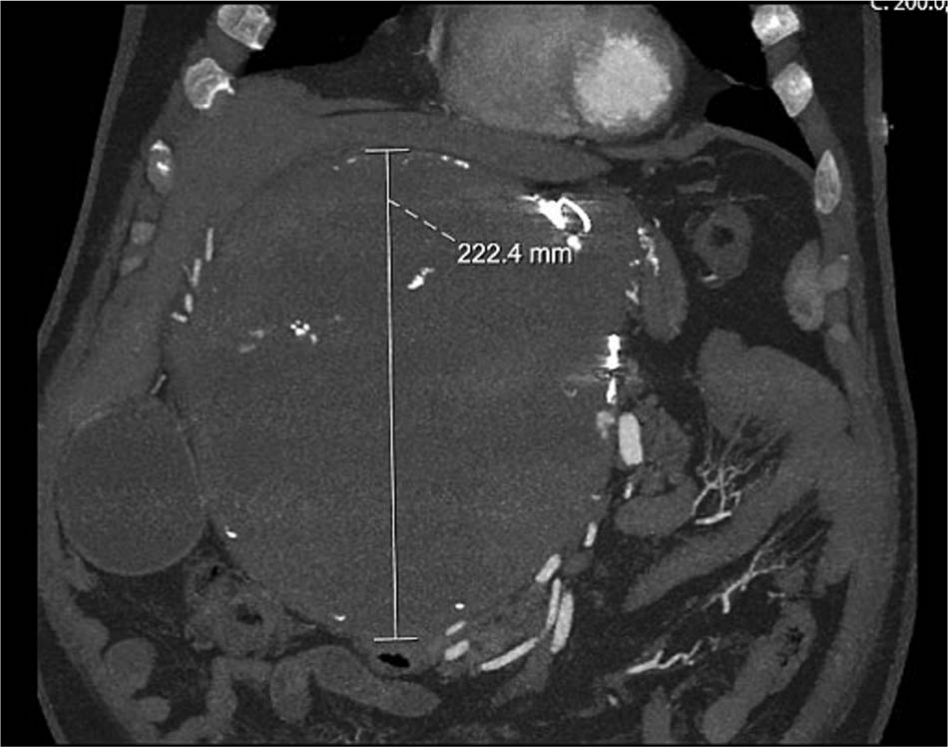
Coronal CT image showing large HAA.
